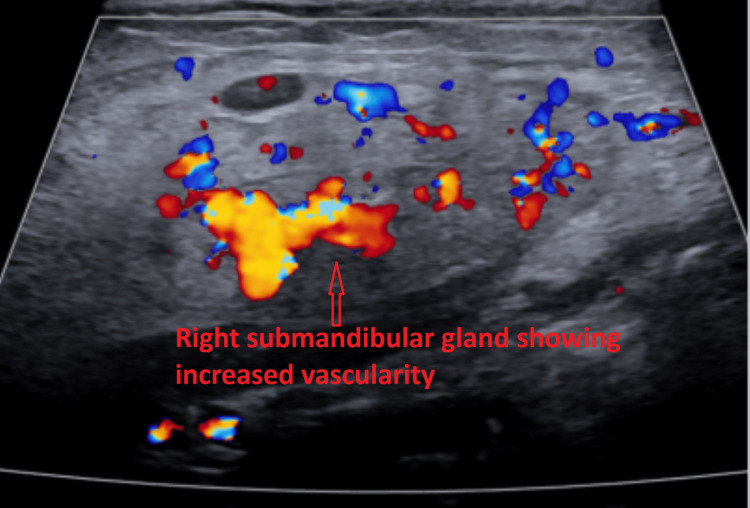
Color Doppler ultrasound image of the transverse view of the right submandibular gland showing increased vascularity
